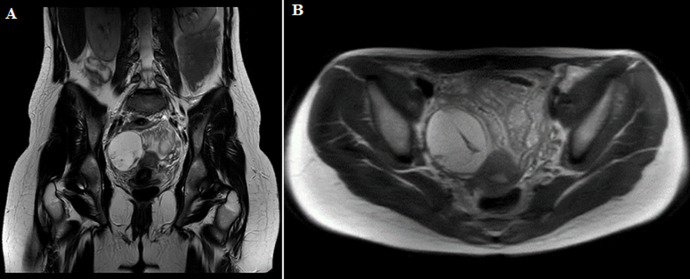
hemorrhagic ovarian cyst features on magnetic resonance imaging; coronal and axial pelvic MRI revealing an ovoid cystic mass, with internal septations and microhemorrhagic elements (6 x 5 x 6 cm)
